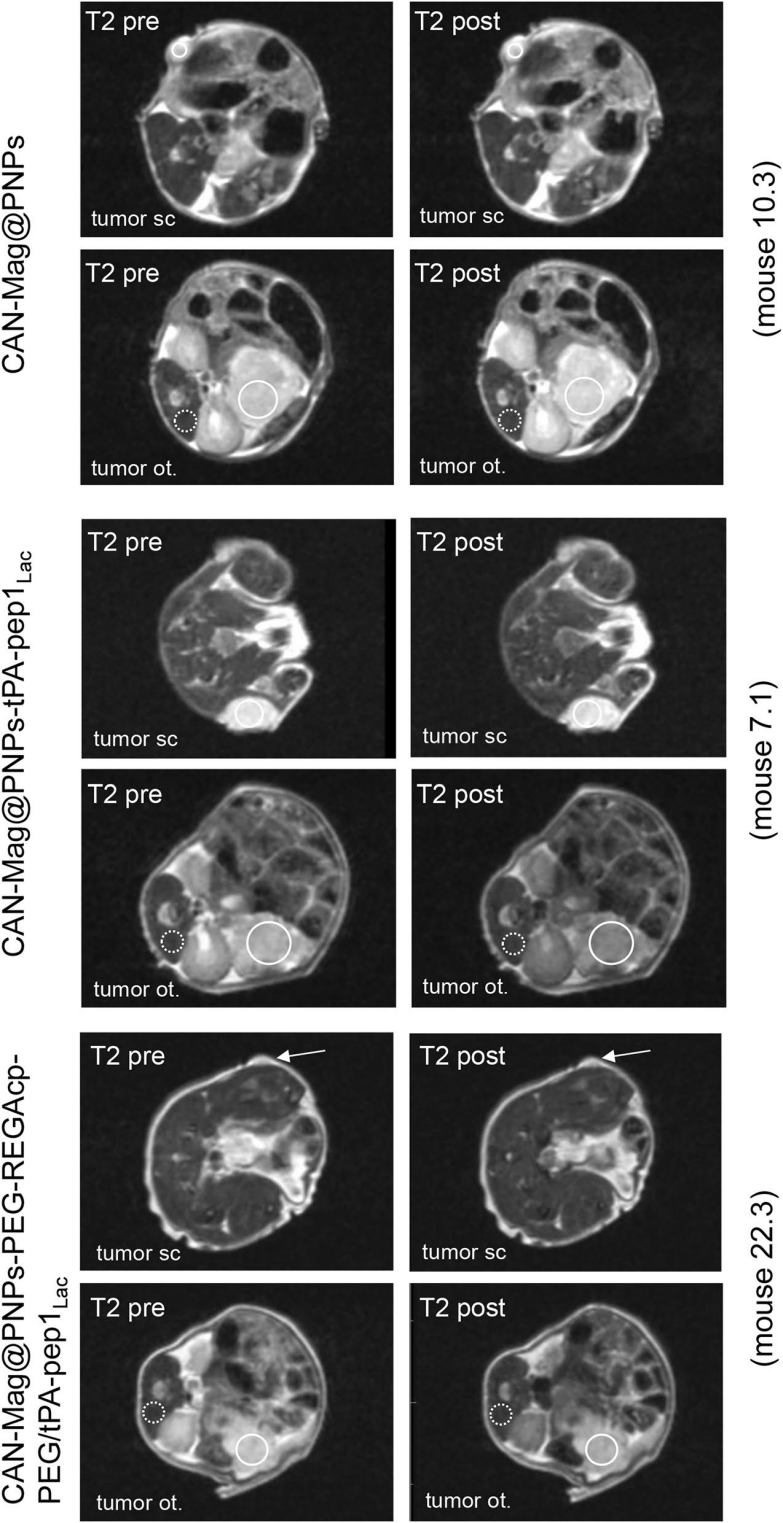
MRI: a minor portion of the injected NPs reach the tumor. Axial presentation of subcutaneous (sc) and orthotopic (ot) Panc-1 tumors T2-weighted images of a single slice before (*left*) and 5 min after injection (*right*) of CAN-Mag@PNPs (*top*); CAN-Mag@PNPs-tPApep1_Lac_ (*middle*); and CAN-Mag@PNPs-PEG-REGAcp-PEG/tPA-pep1_Lac_ NPs injection (*bottom*). The circled tumor areas represents the regions of interest (ROI) used for the analysis of signal intensity loss. The *dashed circle* areas are the muscle areas used as reference ROI. A signal drop (*darker area*) in the s.c. and ot. tumors can be seen after NP application in the T2 weighted images
